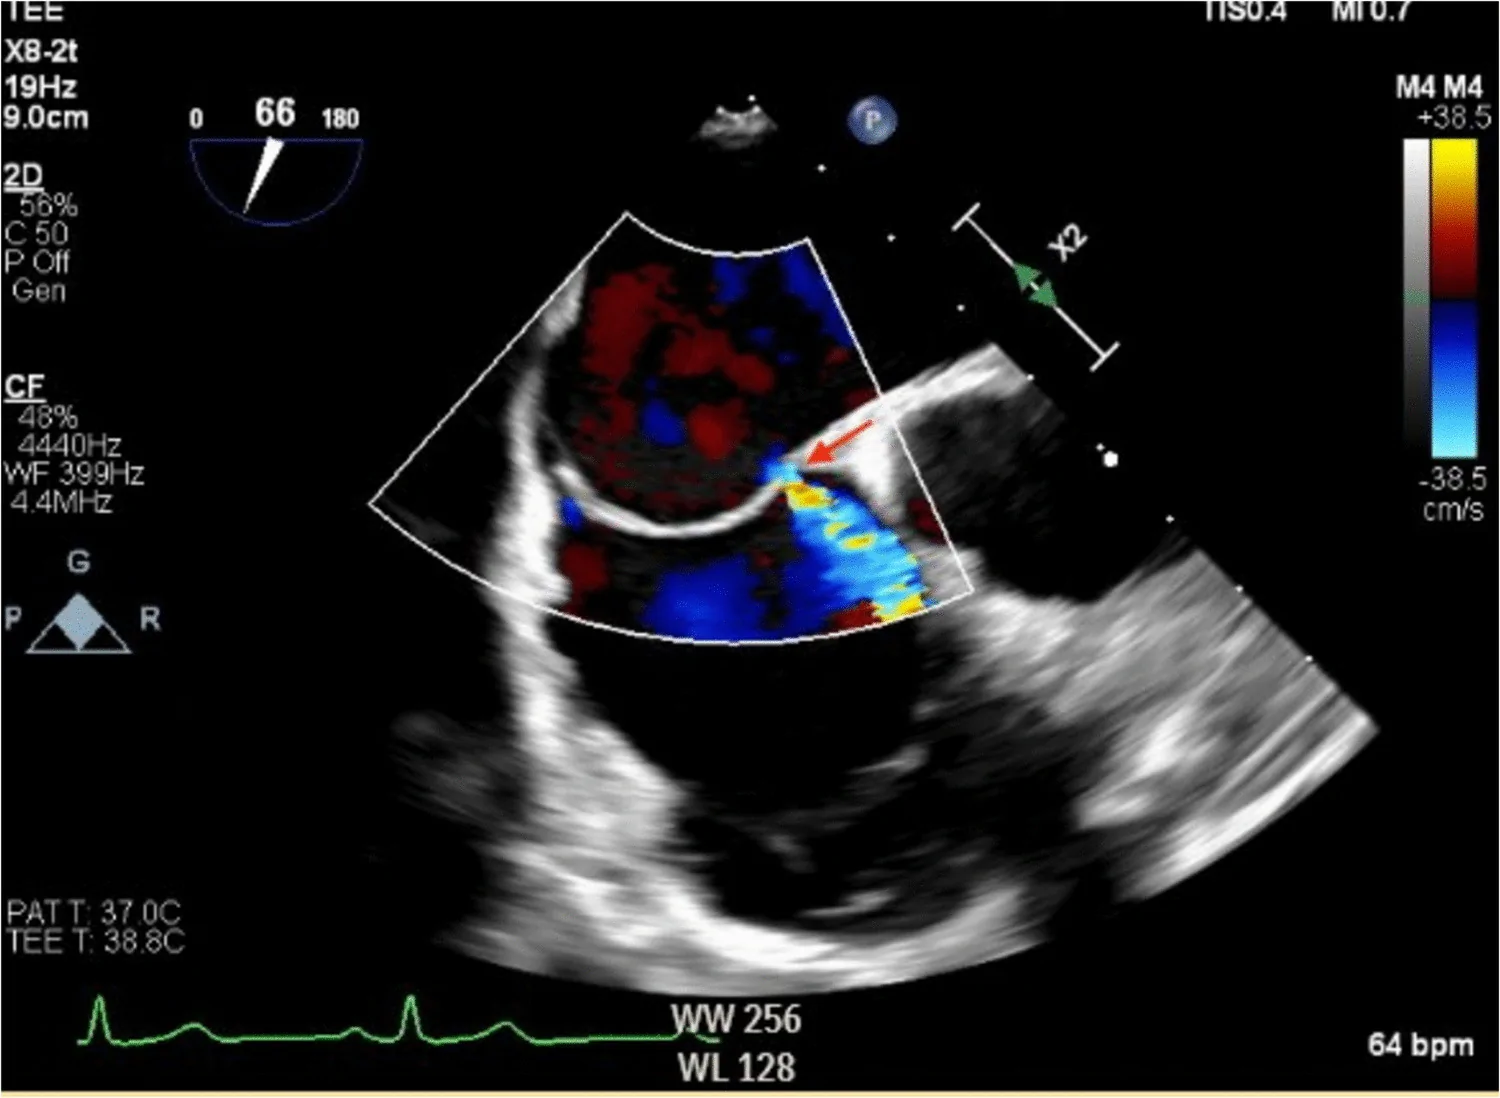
Transesophageal echocardiogram (TEE). Static image from TEE, identifying atrial septal defect with atrial septal aneurysm with turbulent flow (red arrow). Original clinical image from the patient
